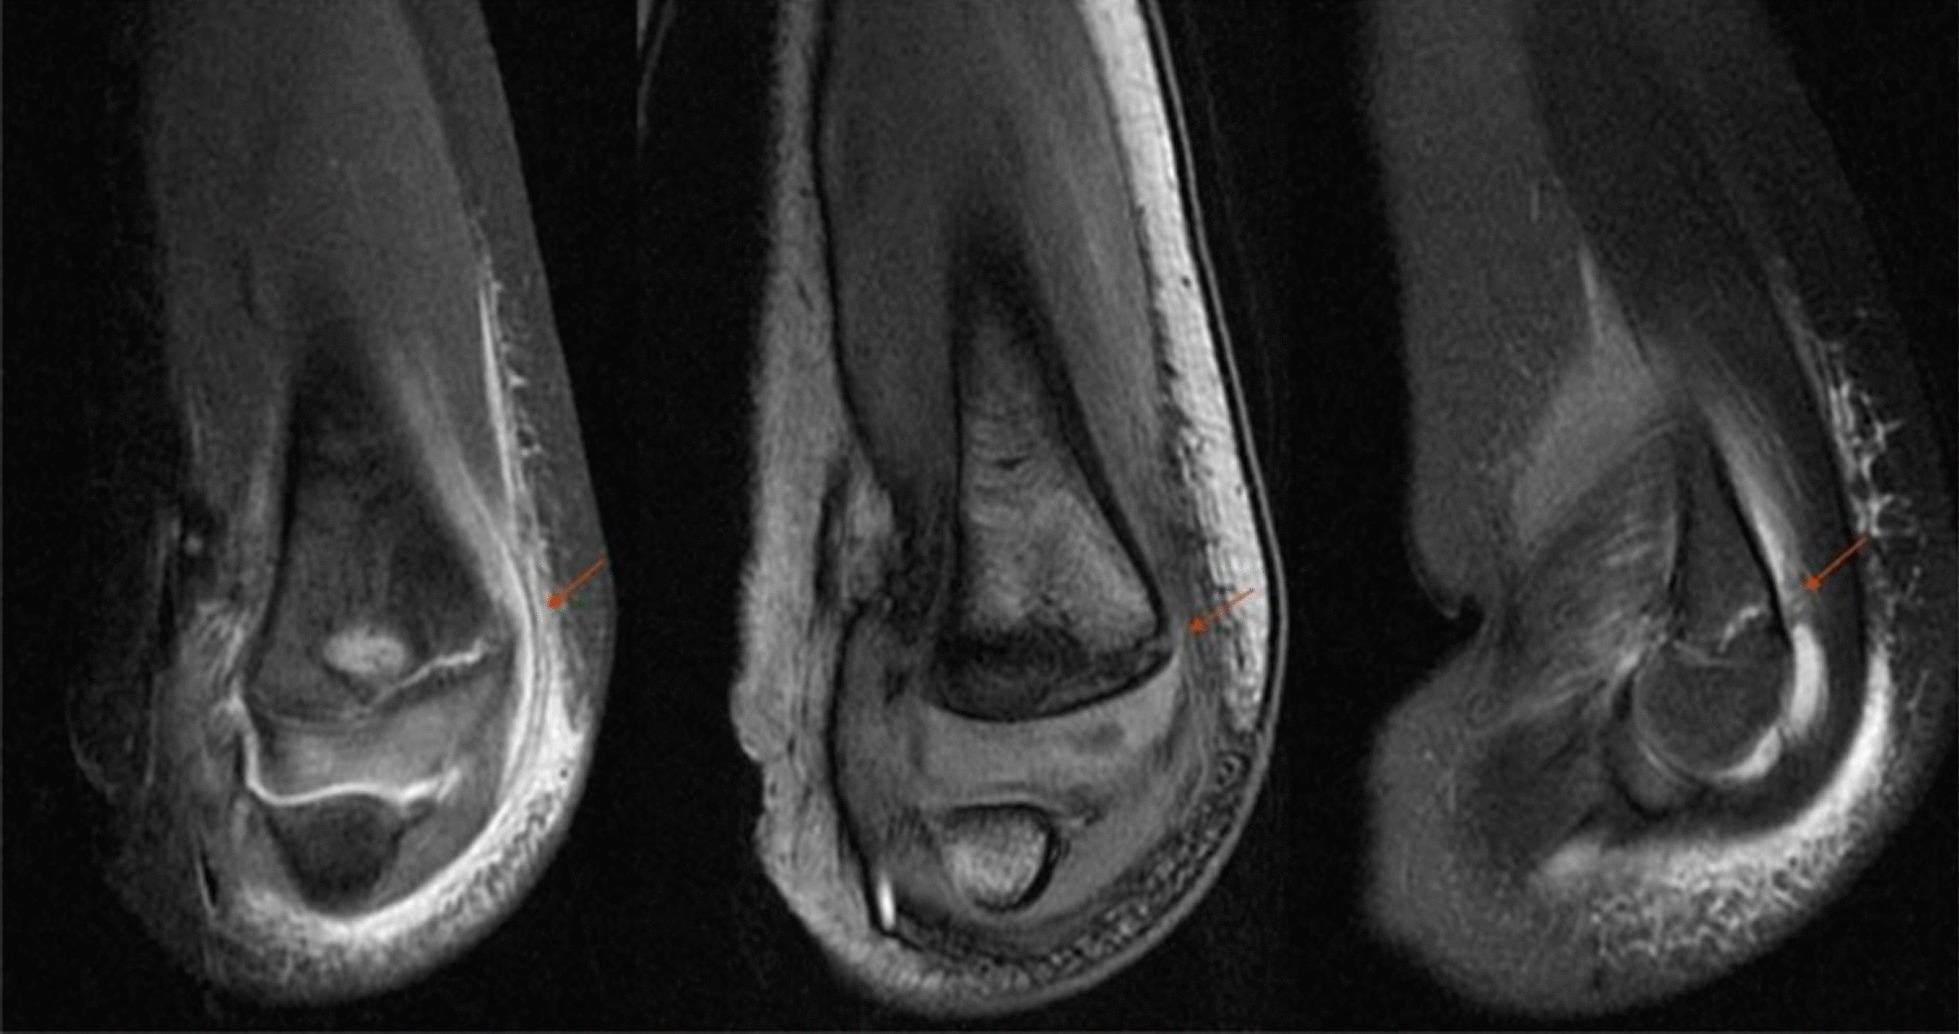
Three years old girl with left elbow HLCF. She was diagnosed undisplaced HLCF by radiograph. MRI showed it was stable fracture and the fracture line was limited to the distal metaphysis of the humerus (Left: coronal FS-T2WI; Mid: coronal 3D-FSPGR; Right: sagittal FS-T_2_WI; The arrow showed the fracture line). HLCF, Humeral lateral condyle fracture
